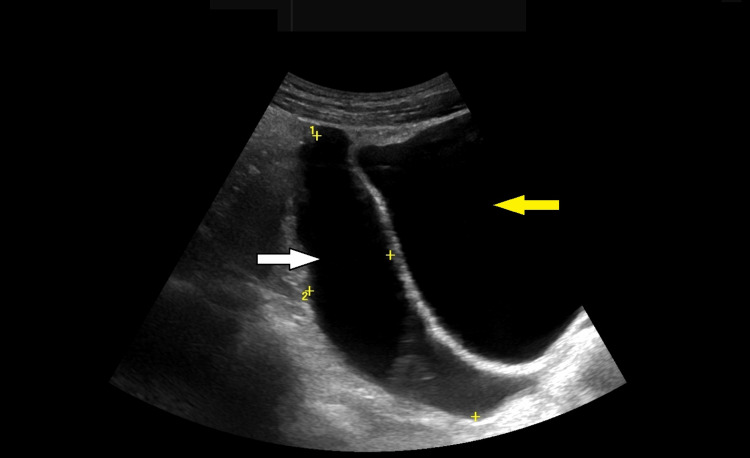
Pelvic ultrasound demonstrating a pelvic collection (denoted by the white arrow) beside the urinary bladder (denoted by the yellow arrow)
